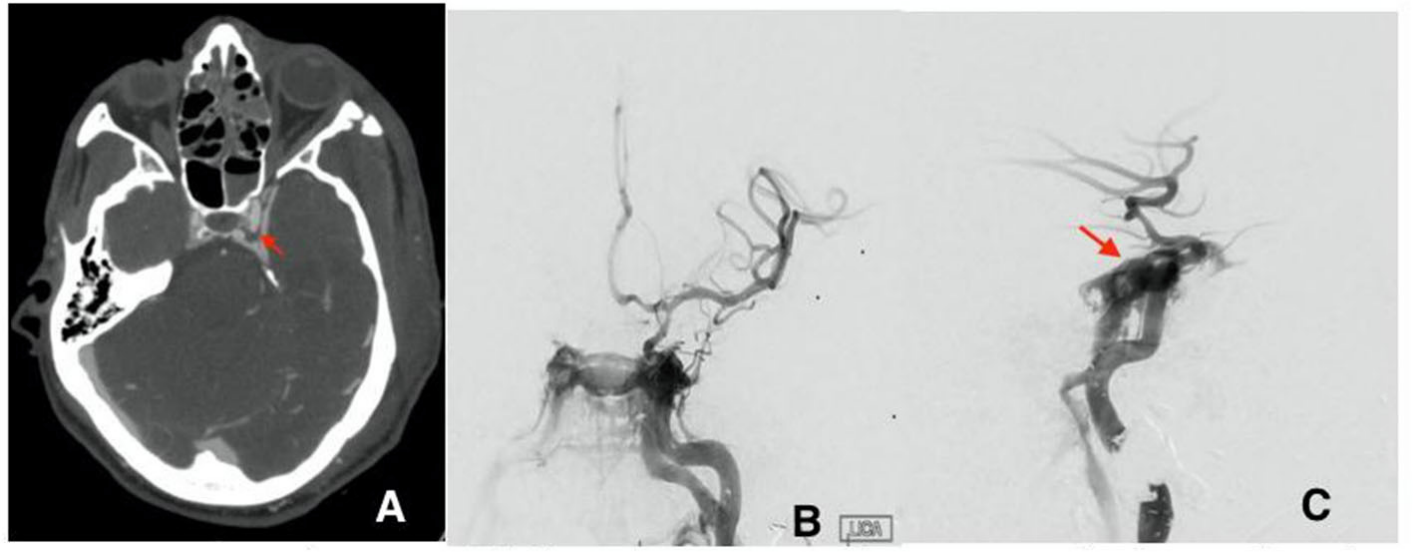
(A) Initial CTA with left cavernous pseudoaneurysm (red arrow). (B) first cerebral angiogram AP view and lateral view (C) showing the direct fistula between the cavernous segment of the left ICA and the left cavernous sinus at the site of ruptured pseudoaneurysm.
